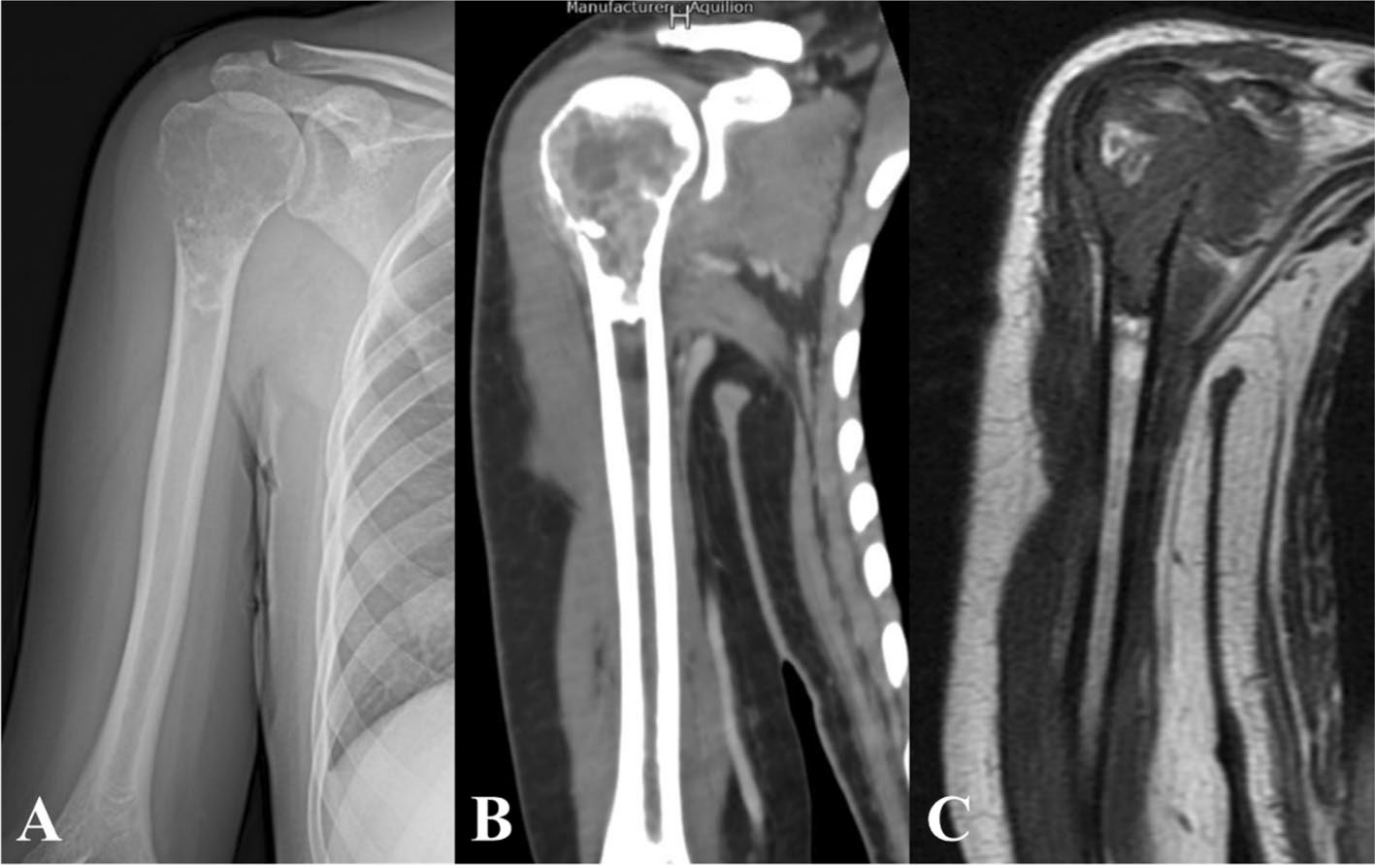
The preoperative humerus radiography (A), CT (B) and MRI (C) of a patient with proximal humerus osteosarcoma. The extent of tumor resection and length of osteotomy was determined according to preoperative imaging examinations.
